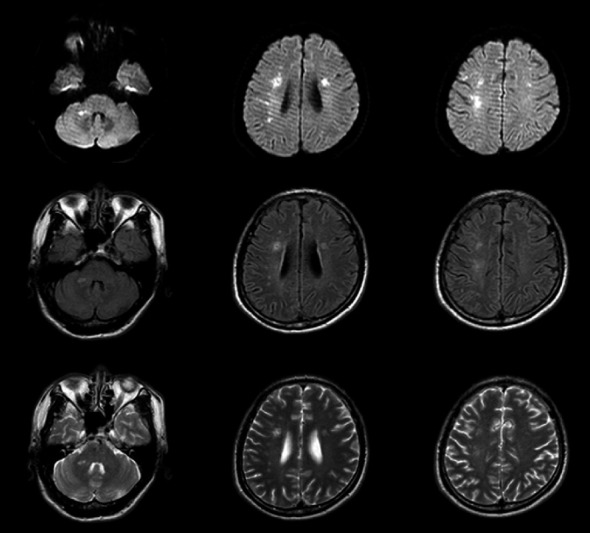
Head MRI (January 25, 2018 in Liaocheng People's Hospital) showed multiple new focus in bilateral semiovale center, corona radiata, and right bridge arm.
